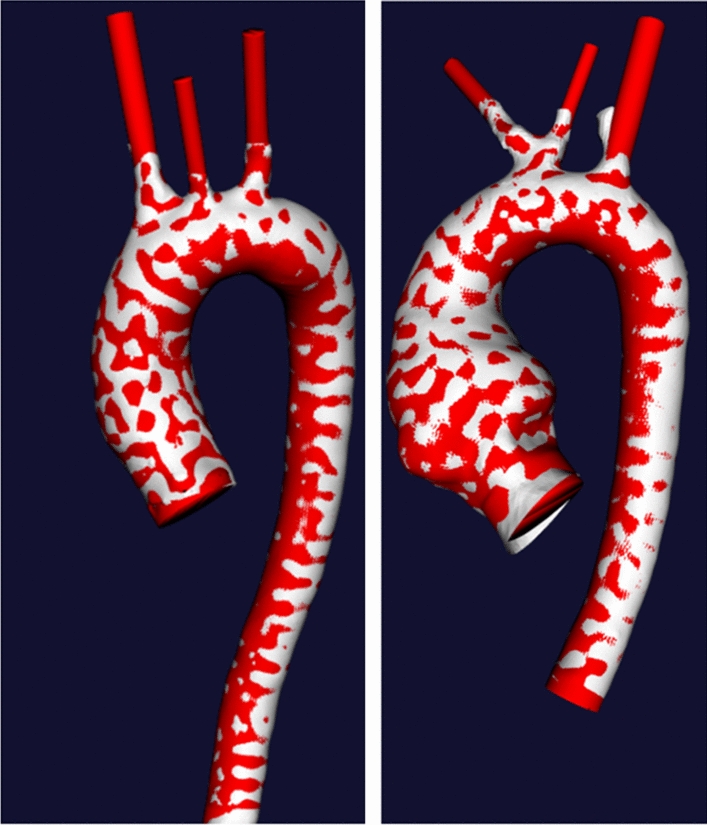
Initial surface obtained from 4D-flow MRI (white) and the geometry after the final step of pre-processing (red) for healthy control (left) and patient (right)
